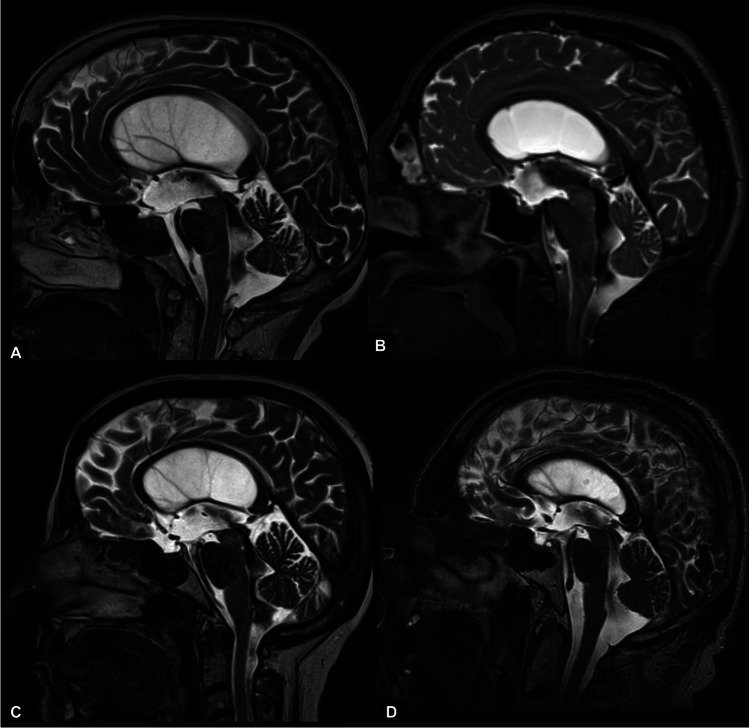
Examples of LOVA in T2-weighted MRI midsagittal view. An enlarged cisterna magna with a patent aqueduct and a turbulent flow is a constant finding (A–D), among other common peculiar features, such as sellar distortion (A, B) and third ventricle floor bulging (A–C). The anatomy of the posterior cranial fossa is distorted as an effect of the cisterna magna enlargement: the folia of the cerebellar vermis are less appreciable and shifted upwards
